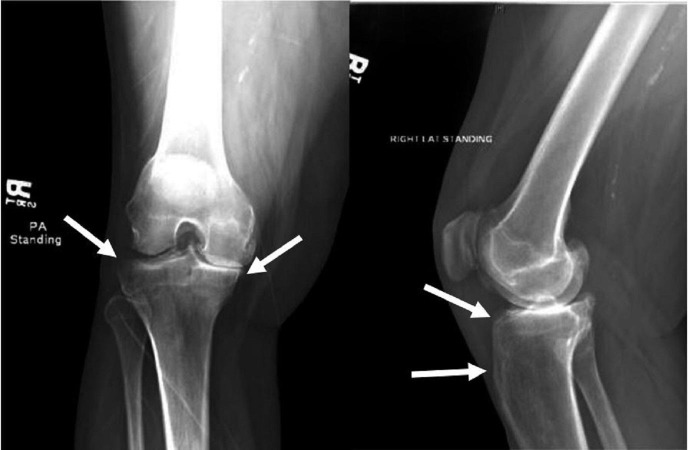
Roentgenogram of right knee. Note the bone expansion and marked sclerosis.
